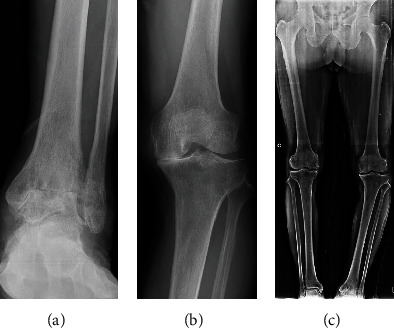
(a) An anteroposterior (AP) radiograph of a 72-year-old male patient who developed Takakura's classification stage 4 osteoarthritic changes of the left ankle that failed to respond to conservative management. (b) An AP radiograph of Kellgren-Lawrence Grade IV osteoarthritic changes in the left knee (c) A preoperative standing AP radiograph of the bilateral lower extremities showed a left hip-knee-ankle (HKA) angle of 14.3°.
